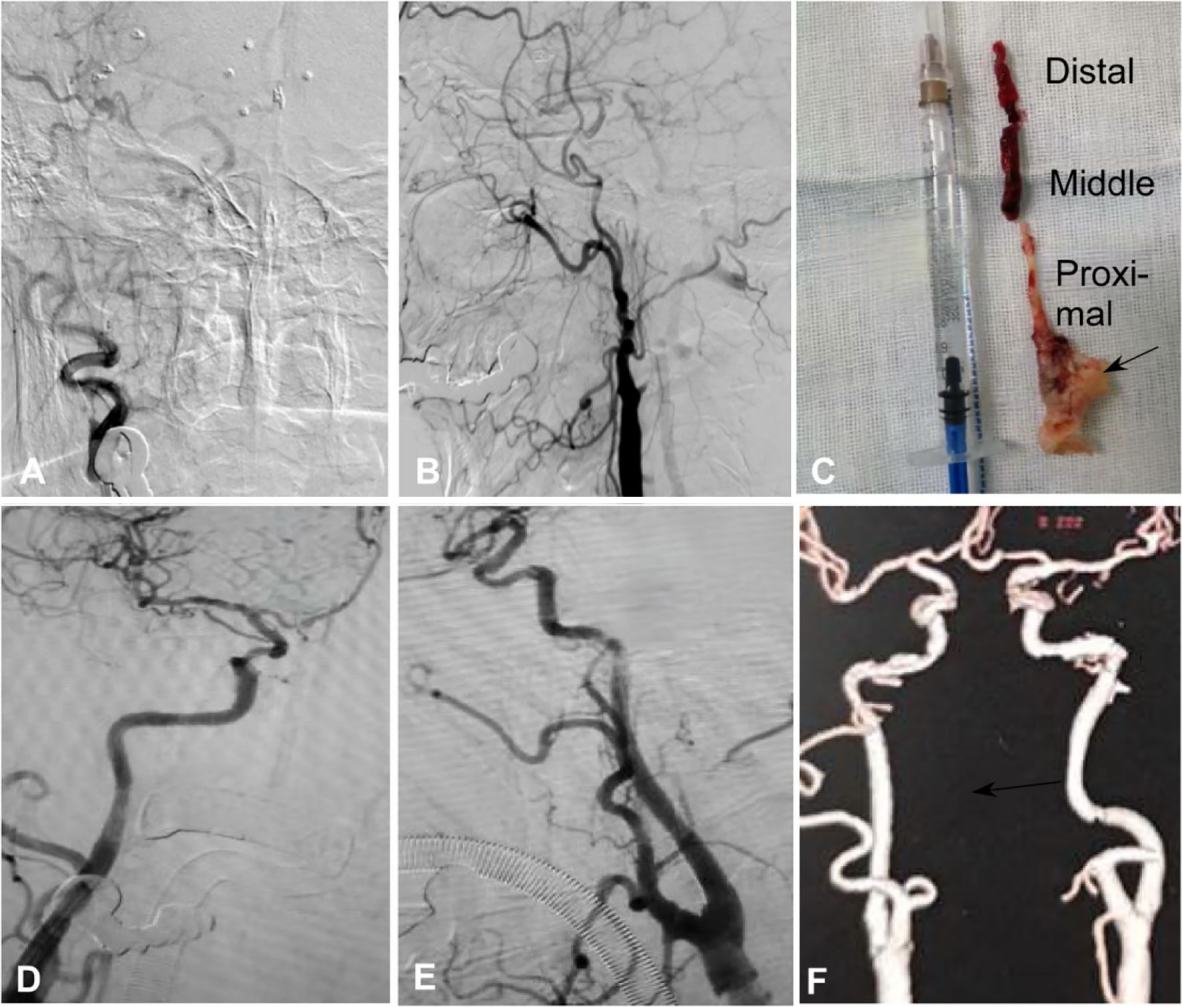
A patient with long-segment occlusion of the right carotid artery. (A and B) Cerebral angiography showed occlusion of the right carotid artery. (C) Carotid endarterectomy was performed to remove the clot occluding the carotid artery. The clot was white organized at the carotid bifurcation and proximal segment of the internal carotid artery (ICA), old dark red in the middle ICA and old red in the distal ICA segment. (D and E) Immediately after stenting with two stents, the carotid artery was patent. (F) Six-month follow-up with computed tomographic angiography demonstrated patient carotid artery.
